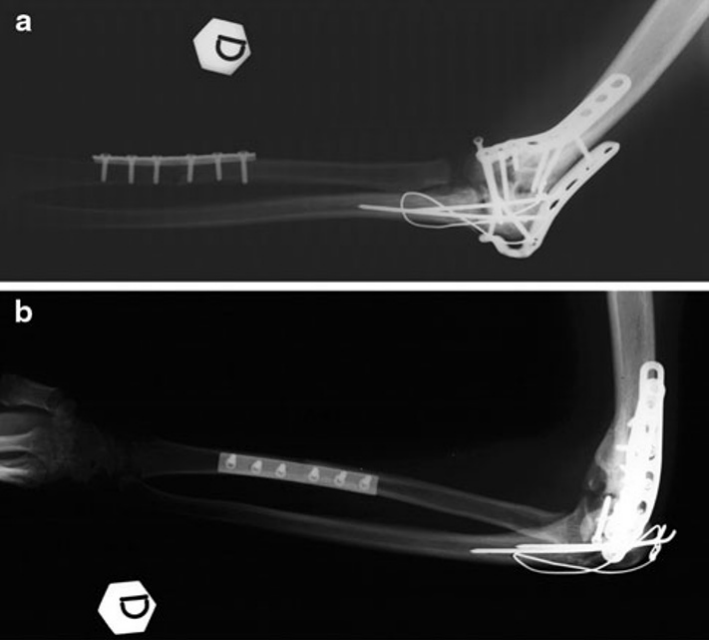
Plain radiographs (a, b) showing the healing of fractures 1 year after the first floating elbow injury
